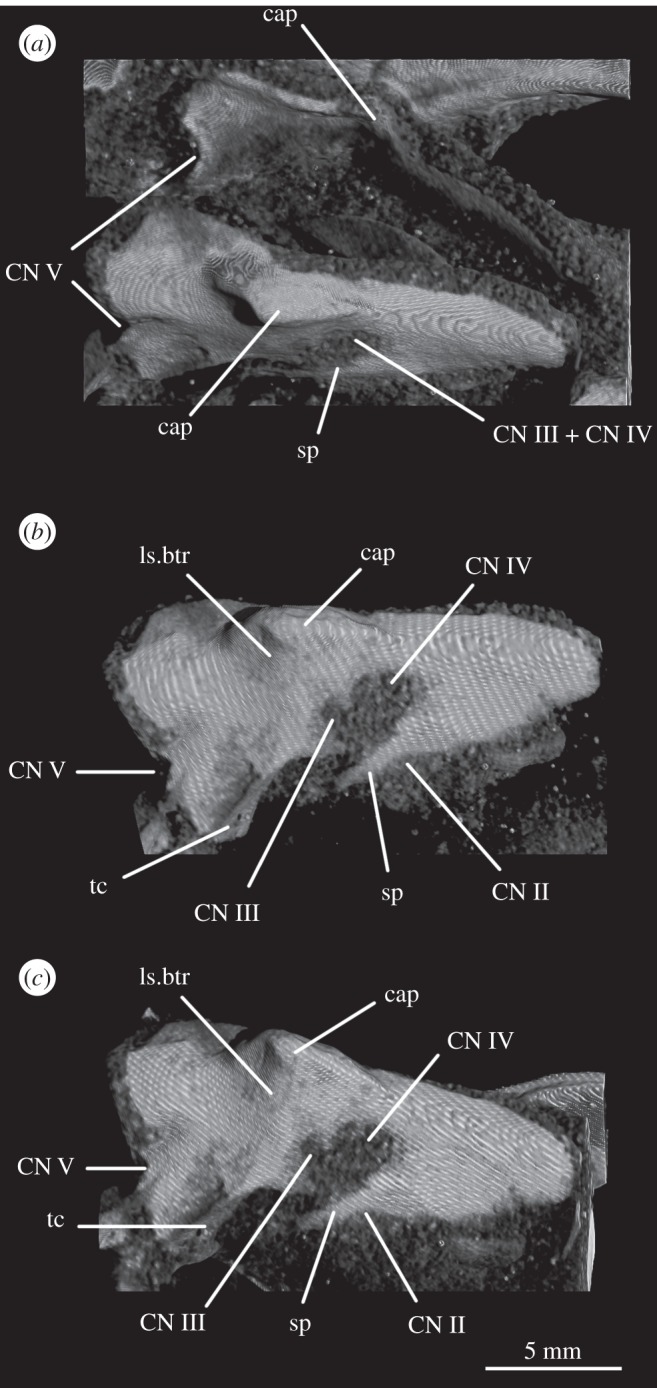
CT reconstructions of laterosphenoid(s) of SAM-PK-5867 in (*a*) right dorsolateral, (*b*) right lateral and (*c*) right ventrolateral views. For abbreviations, see table 1.
